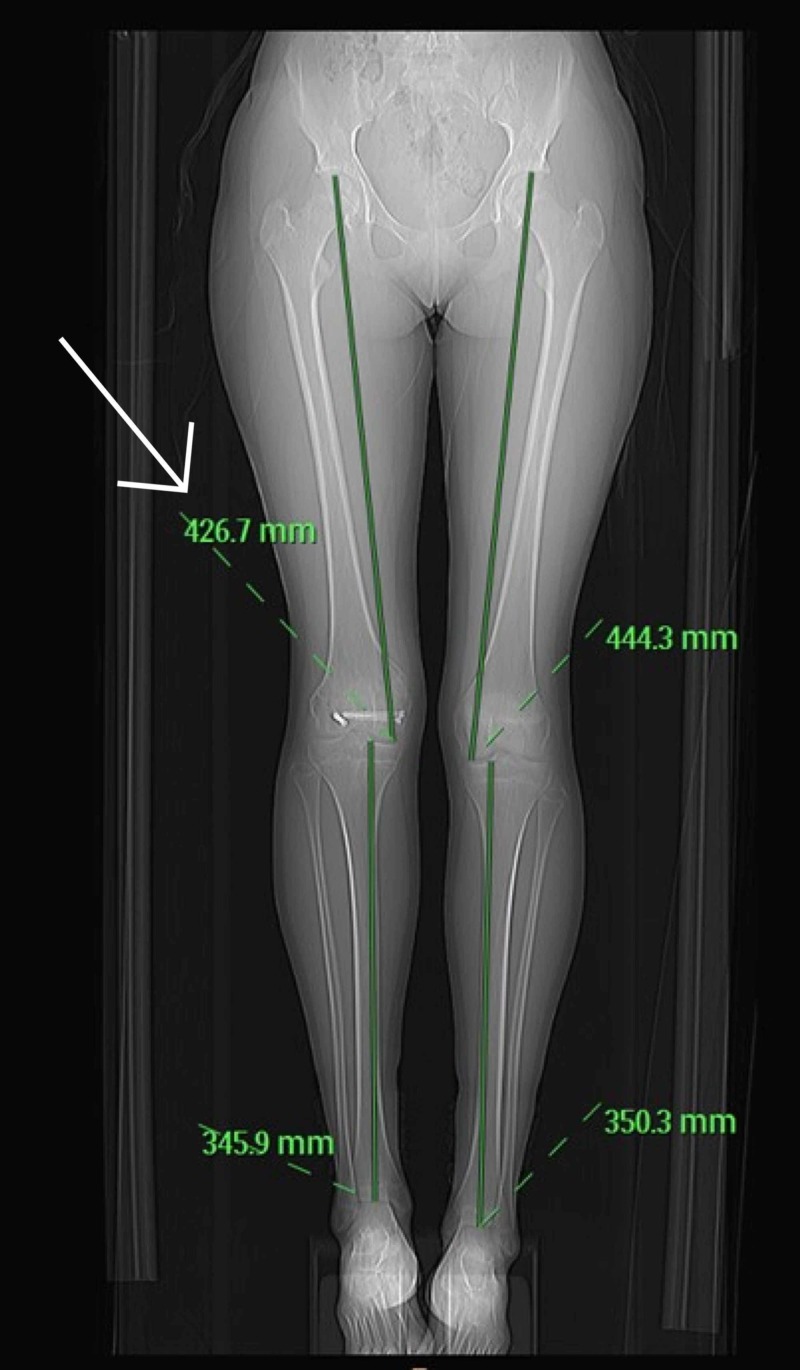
Computed tomography scannogram performed which demonstrated a limb length discrepancy
